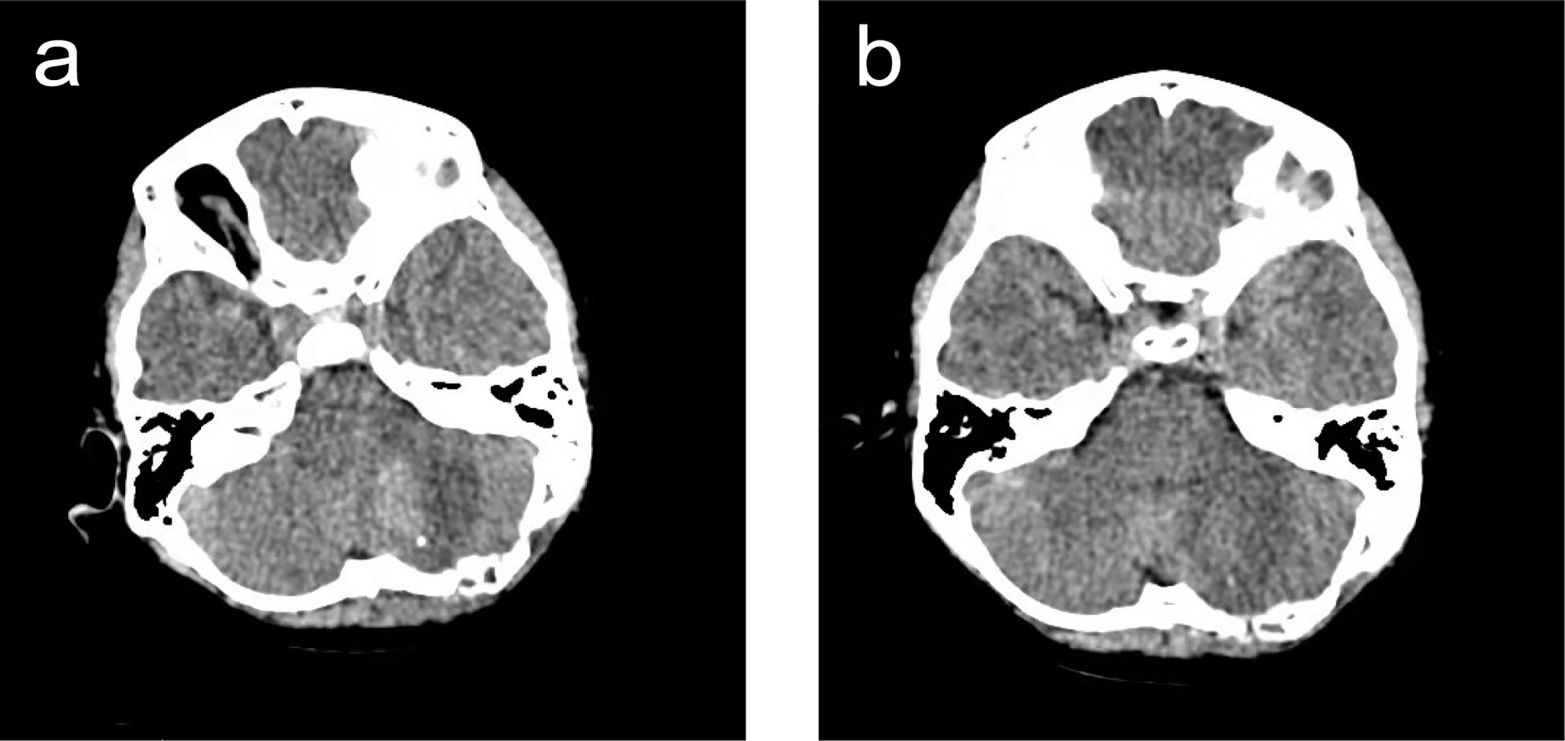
Radiological evaluation of YST post operation. CT scanning of the residual tumor on day 7 (A). and two weeks after operation (B). The size of the residual tumor significantly decreased. Patchy hyperdense shadow was seen in the left cerebellar hemisphere, surrounded by patchy low-density edema. No obvious abnormalities were found in the ventricle or cisterns. Displacement of middle structure was not found.
